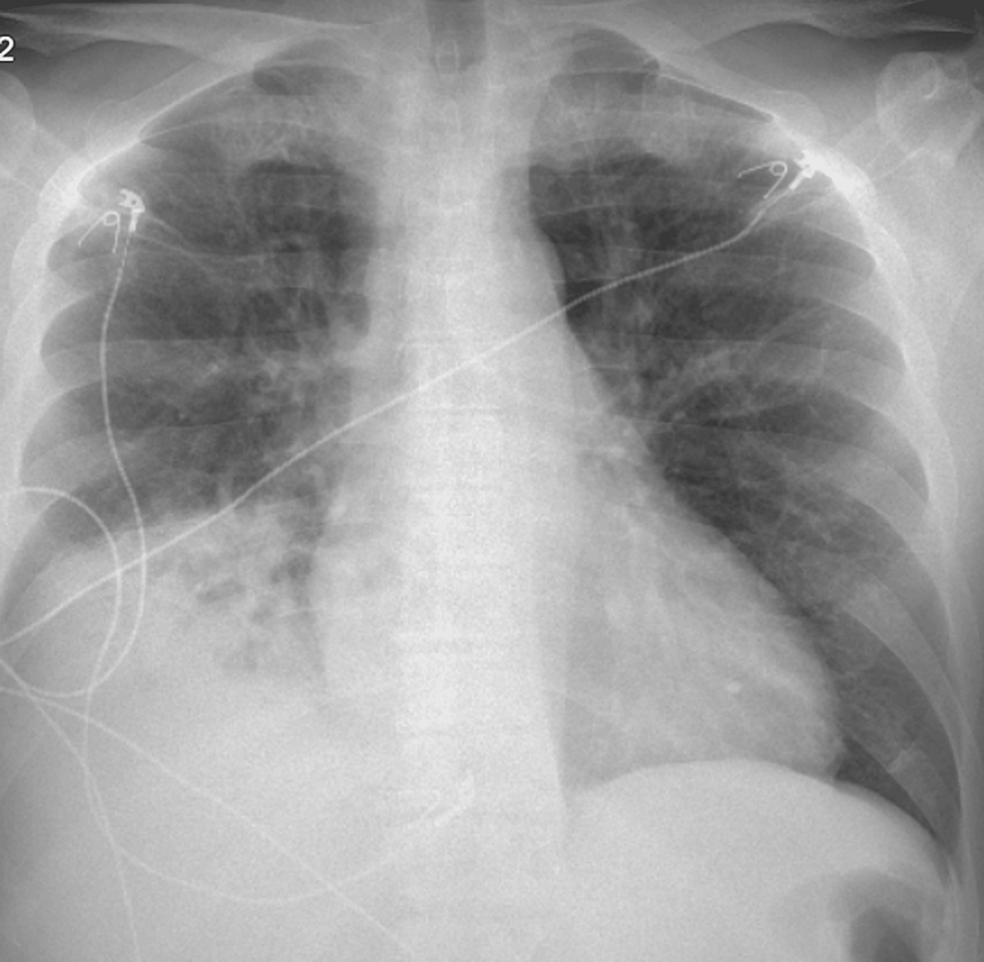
AP chest X-ray showing hazy opacification of the right lower lung field; areas of lucency in the medial margin of the consolidation. AP: Anteroposterior
